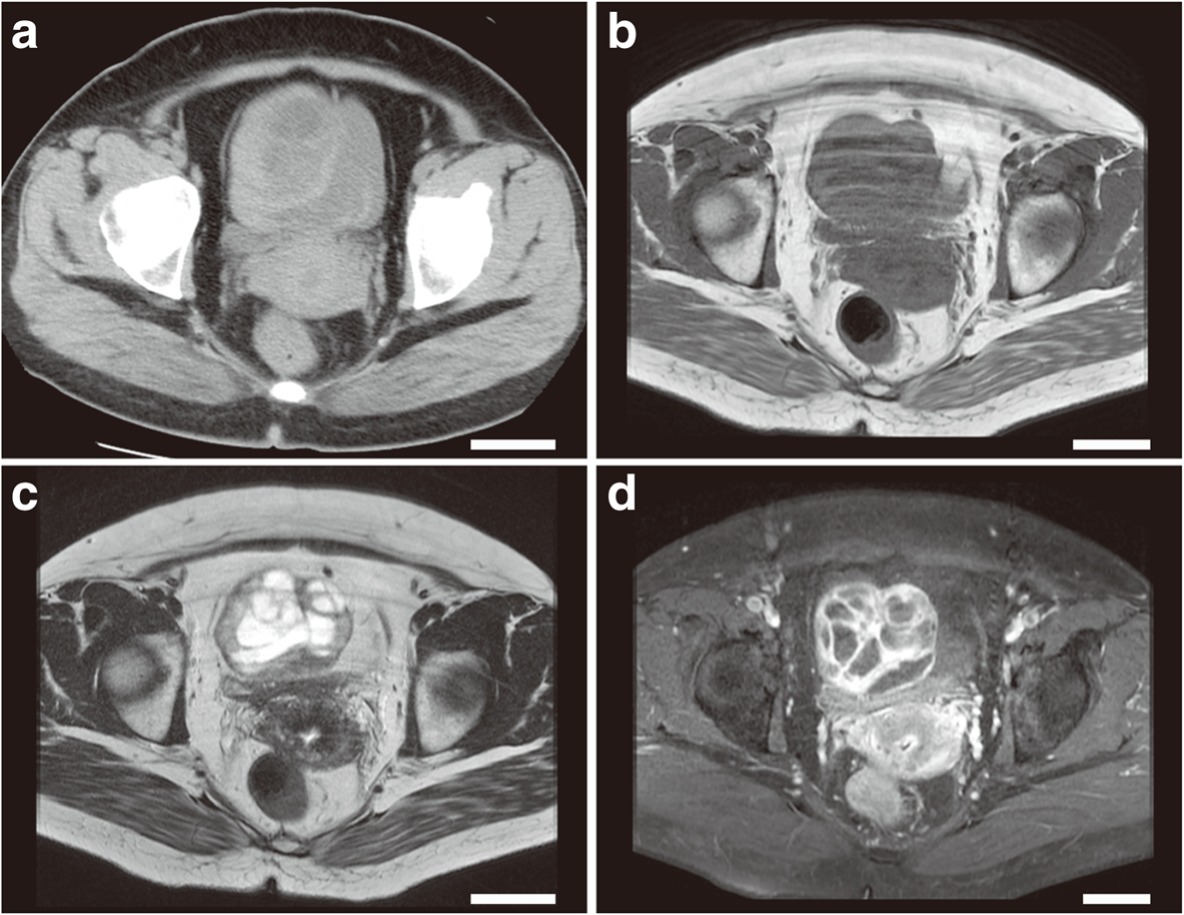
Radiographic images of the tumor. (a) An axial non-enhanced computed tomography image. A nodular lesion was seen adjacent to the uterus. The mass had low-density areas, and the peripheral and septal portions were isodense relative to the uterus. (b–d) Magnetic resonance images in the axial plane. The tumor had an intermediate to low signal intensity on a T1-weighted image; (b) a high signal intensity, particularly of the cavities, on a T2-weighted image (c); and a multilocular enhancement pattern on a contrast-enhanced, fat-suppressed T1-weighted image (d). The *white bars* represent 5 cm
